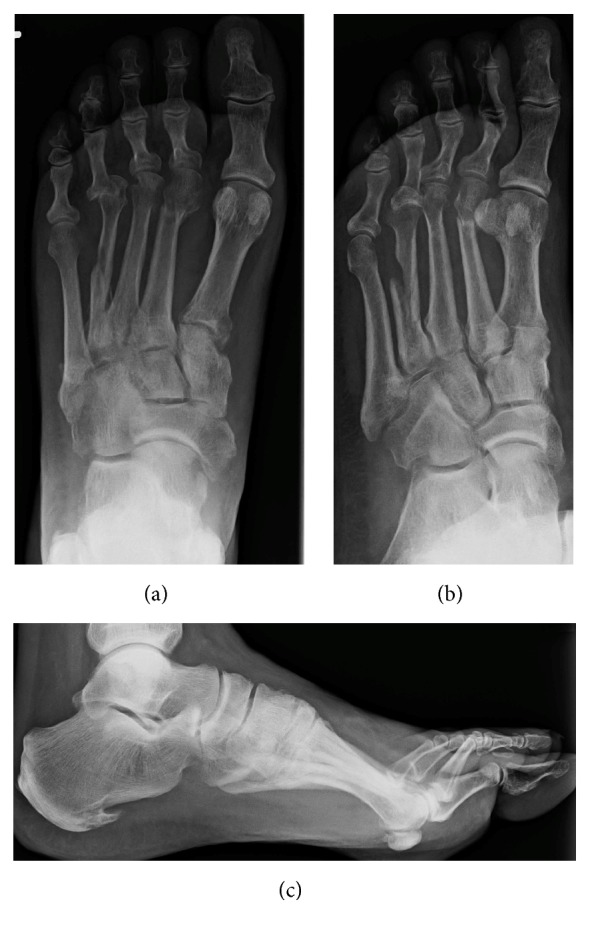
Preoperative dorsoplantar (a), oblique (b), and lateral (c) radiographs of the left foot showing malunion at fracture site, resulting in a lengthened fourth MT, and a dorsal dislocation at the fourth MTP joint.
